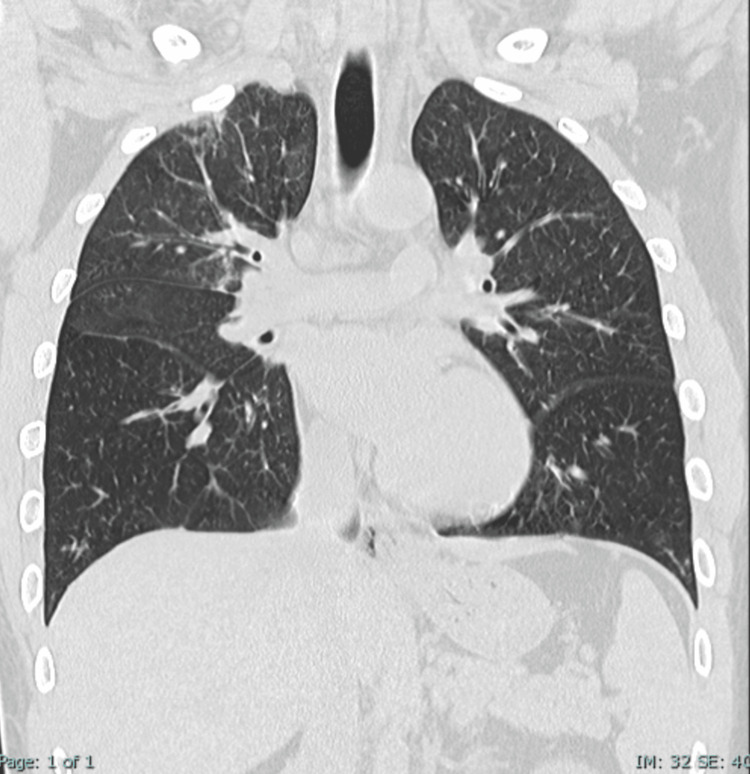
Follow-up chest computed tomography (CT) on hospital Day 10 demonstrating marked resolution of the bilateral pulmonary infiltrates.
